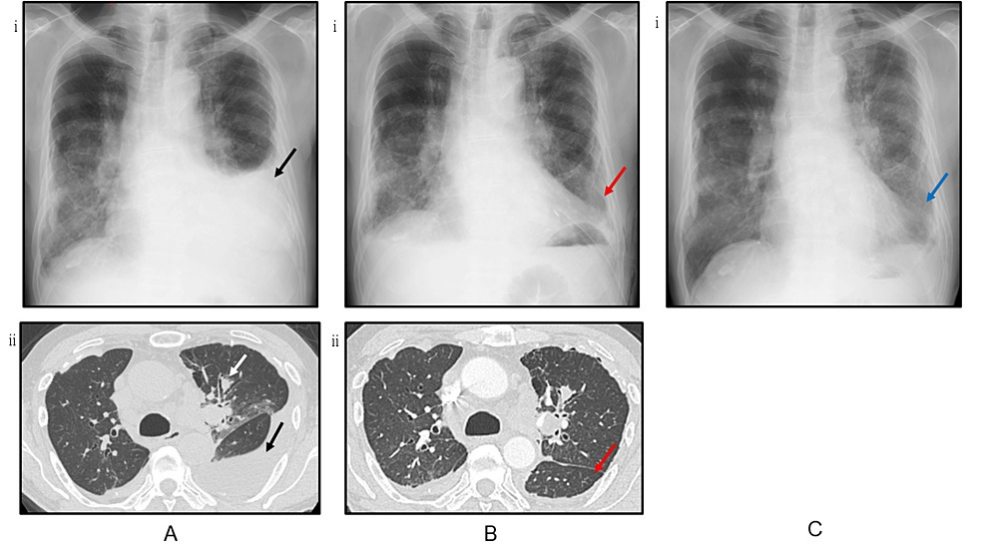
Radiological course before pembrolizumab administration. A. Chest X-ray (ⅰ) and CT (ⅱ) at first visit showed a nodule in the left upper lobe (white arrow, image ii) with bilateral pleural plaque. Left lung pleural effusion was also observed (black arrow, image i). B. After drainage, chest X-ray (ⅰ) and CT (ⅱ) showed that the left lung pleural effusion had disappeared (red arrows in images i and ii). C. Chest X-ray 12 days after pleurodesis showed a slight ground-glass opacity on the same side as pleurodesis (blue arrow).
